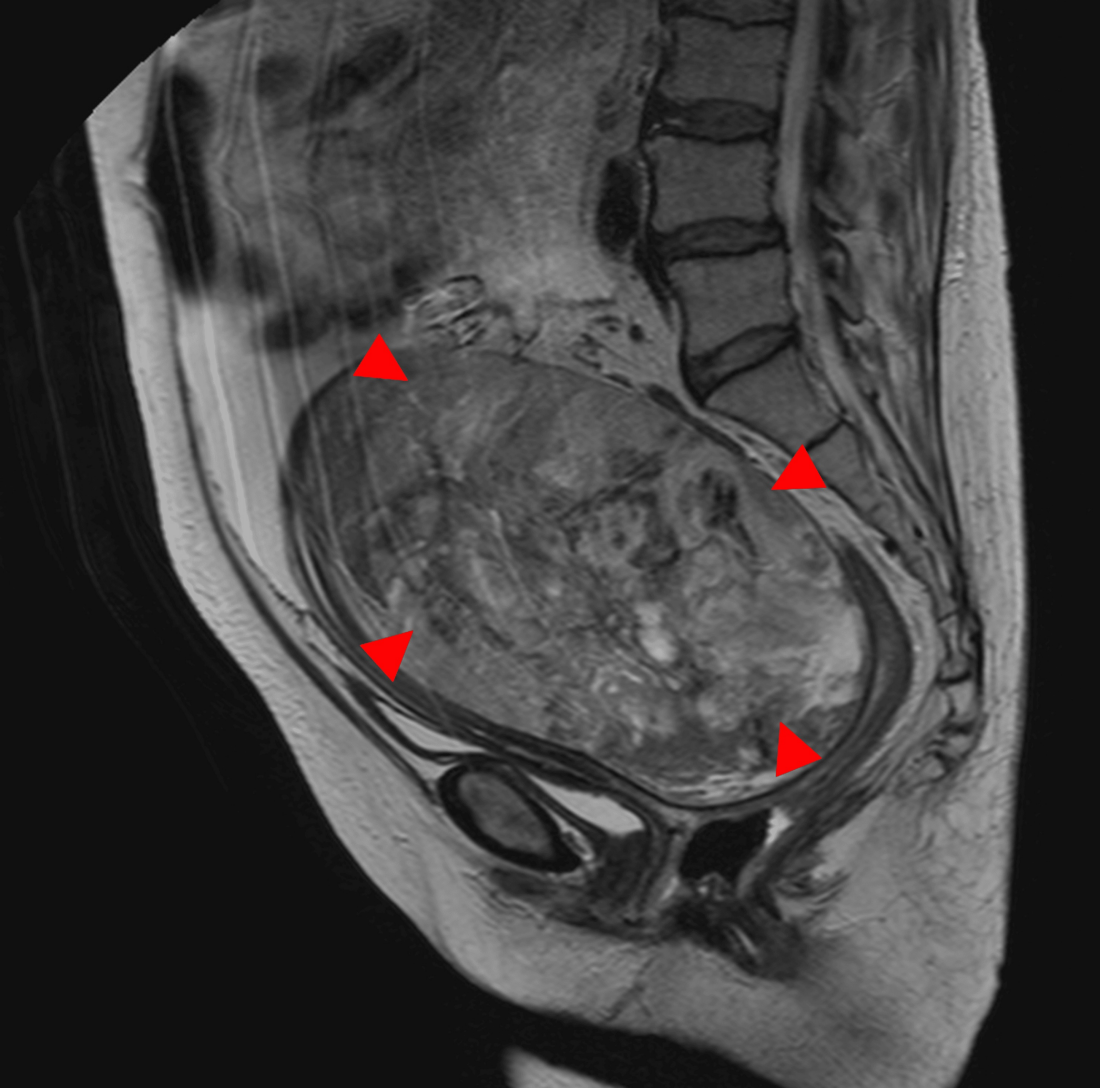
This is the same patient as shown in Figure 11. On the sagittal T2-weighted image, the solid component demonstrates heterogeneous signal intensity with a layered appearance, consistent with the mille-feuille sign (arrowhead).
